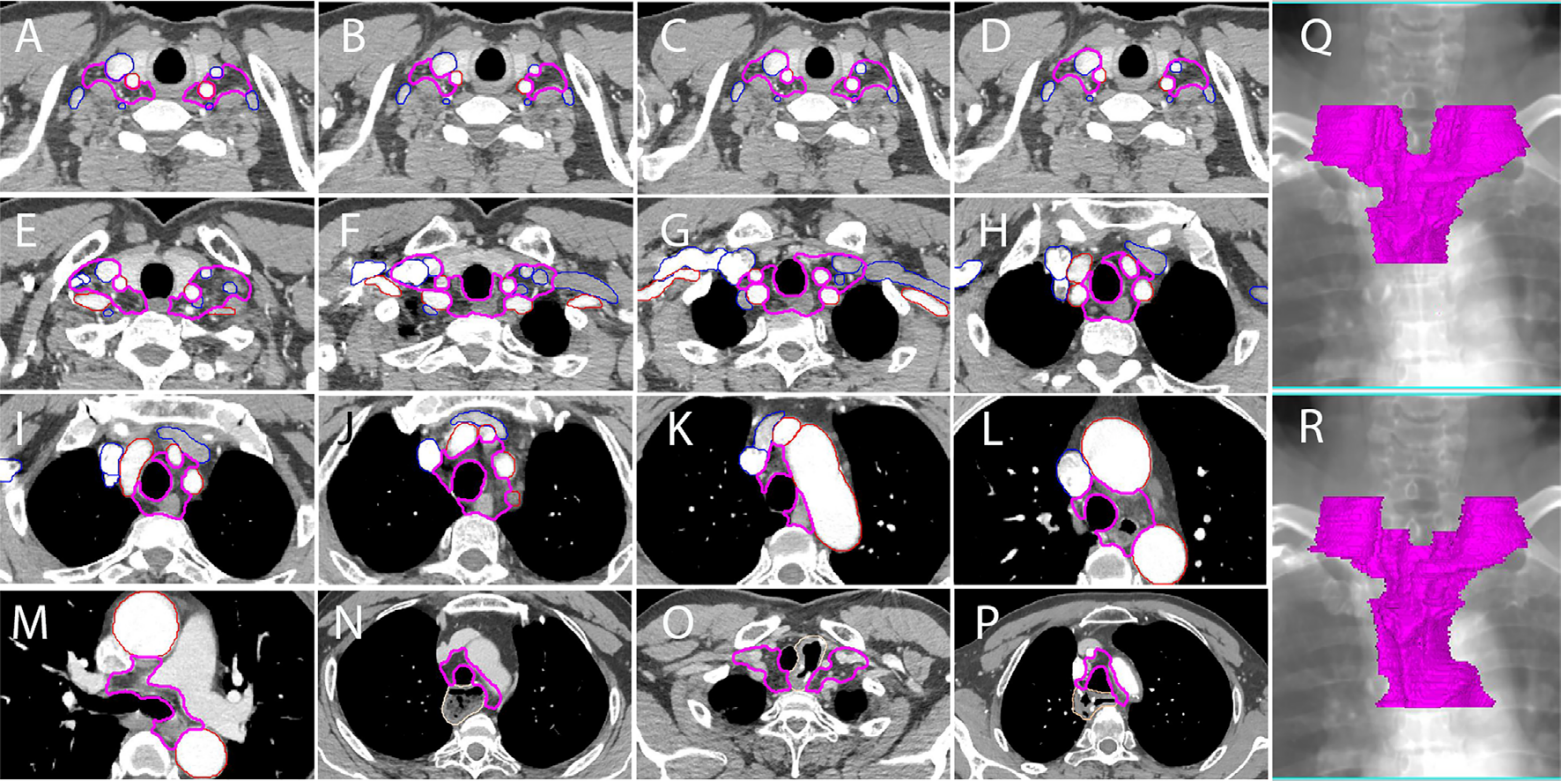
A proposed delineation of CTVnd in lower neck and upper mediastinum for PORT of TESCC. Pink lines: CTVnd; red lines: arteries; blue lines: veins. (A, C) at the level of the cricothyroid membrane (upper TESCC); (B, D) at the level of the cricothyroid membrane (middle and lower TESCCs); (E) at the level of the upper margin of subclavian arteries; (F) at the level of the lower margin of the horizonal part of subclavian arteries; (G) at the level of the lower margin of thyroid; (H) at the level of the upper margin of innominate artery; (I) at the level when the innominate artery is closely contact with the trachea; (J) at the level of the upper margin of aortic arch; (K) at the level when the aortic arch is closely contact with the trachea; (L) at the level of the upper margin of azygos vein (middle and lower TESCCs); (M) the level of tracheal carina (middle and lower TESCCs); (N) stomach in posterior mediastinum; (O) anastomosis in the cervicothoracic junction; (P) anastomosis in the level of aortic arch; (Q) reconstructed CTVnd on the template CT for upper TESCC; (R) reconstructed CTVnd on the template CT for middle and lower TESCCs.
